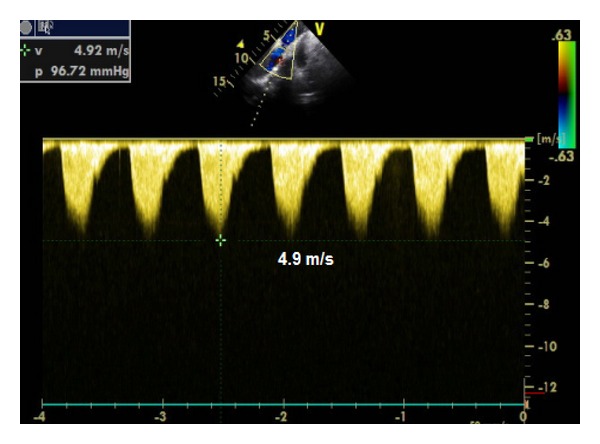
A Doppler echocardiographic gradient was 96 mmHg.
